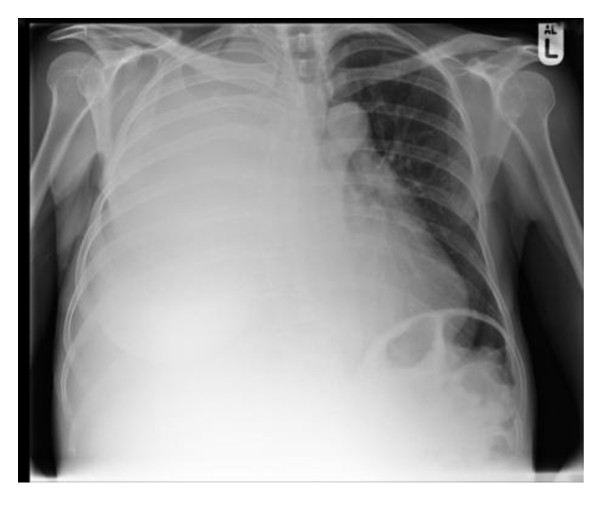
X-ray of patient's chest revealing a right-sided pleural effusion.
